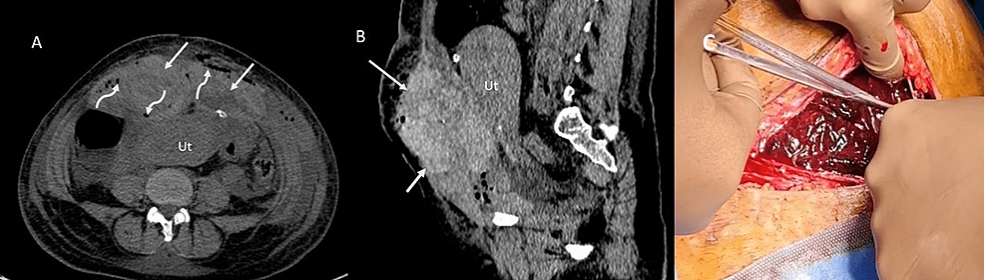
Non-contrast axial (A) and sagittal (B) CT images showing a large hyperdense and heterogeneous lesion (arrow) measuring 14x 4x 15.1 cm (approx. volume 1538 cc) involving bilateral rectus abdominis muscles suggestive of a large hematoma. The lesion is bulging posteriorly with peritoneal extension and mass effect over the uterus (Ut). Note the presence of multiple air foci within the lesion (curved arrows). Intraoperative pic showing large RSH in C. RSH: rectus sheath hematoma
